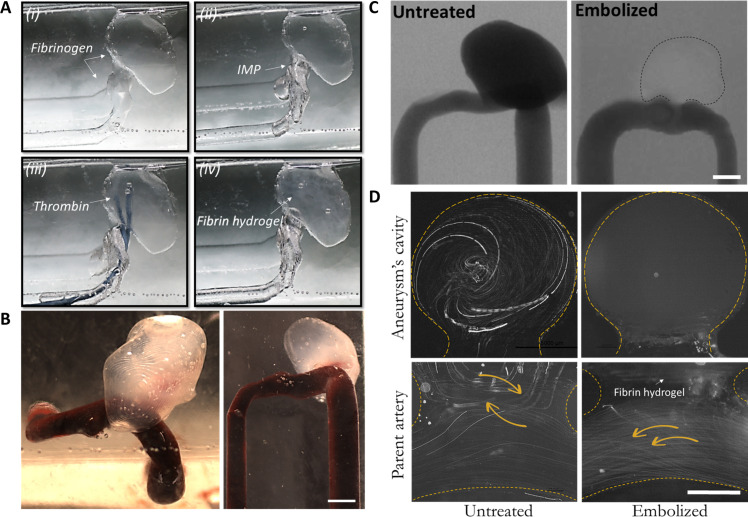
Embolization of surface tension–isolated aneurysms. (A) Time-lapse imaging of the fibrin hydrogel embolization procedure performed in an in vitro aneurysm model using air IMP for the isolation. Scale bar, 5 mm. (B) A fibrin hydrogel embolized aneurysm model following IMP isolation and treatment. Blood is perfused through the parent artery while not entering the fully embolized aneurysm (scale bar, 5 mm). (C) CT angiography images of untreated (left) and the fibrin embolized (right) reconstructed aneurysm model showing full embolization of the aneurysm via the surface tension isolation method where no contrast agent is observed in the cavity. Scale bar, 5 mm. (D) Images of pathlines of 10-μm polystyrene particles flowing under physiological flow through the parent artery entering the aneurysm in the untreated aneurysm (left) while not showing any flow into the fibrin hydrogel embolization aneurysm following IMP isolation and treatment (right). Scale bar, 2 mm.
